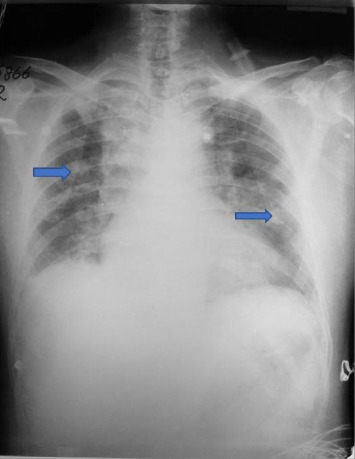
Case 1: Chest radiograph showing bilateral confluent alveolar lesions (arrows) suggestive of severe pulmonary hemorrhagic syndrome.
